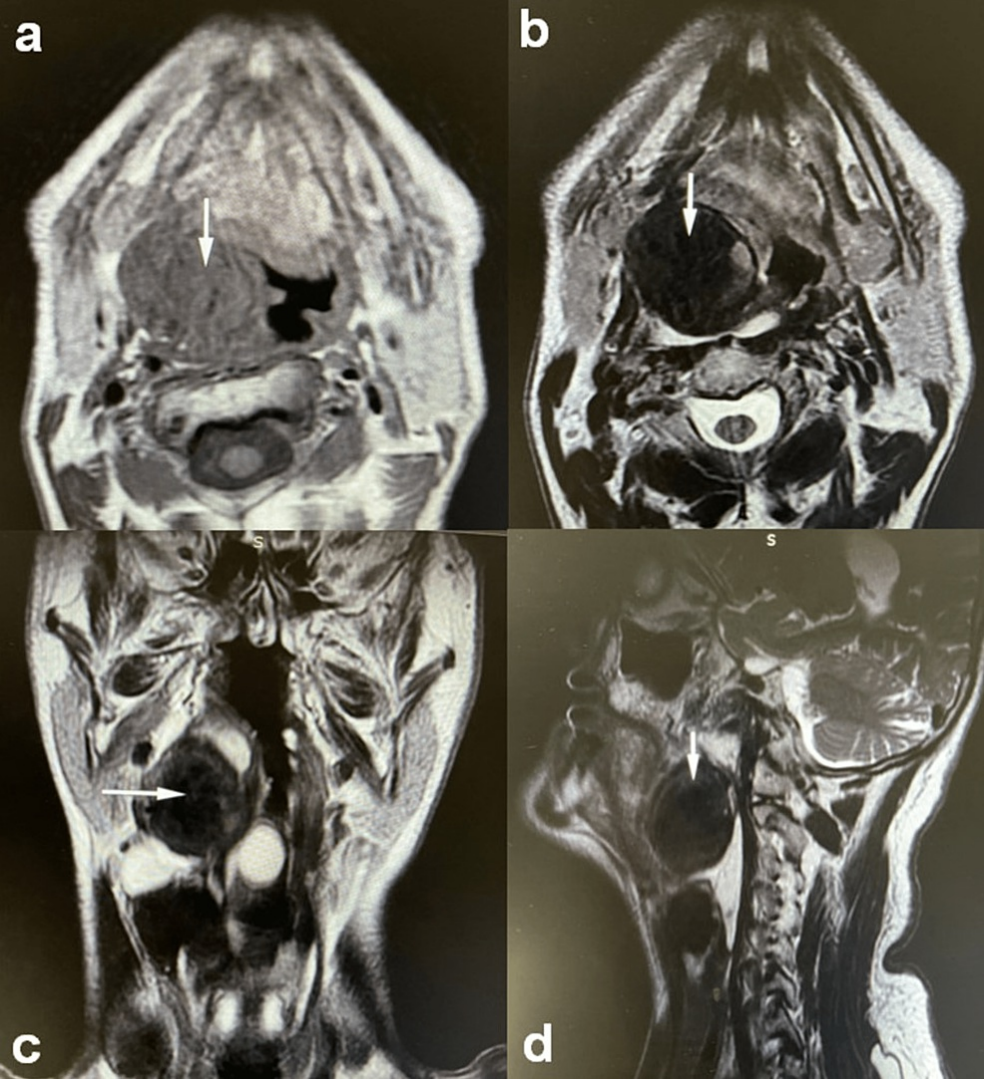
The MRI depiction of a supraglottic laryngeal mass MRI. A growth (white arrow) (5.3cm x 3.9cm) in the supraglottis with inhomogeneous paramagnetic contrast agent uptake and repulsion of adjacent anatomical structures. a. Axial (T1W1 sequence), b. Axial (T2W1 sequence), c. Coronal (T2W1 sequence), d. Sagittal (T2W1 sequence)
